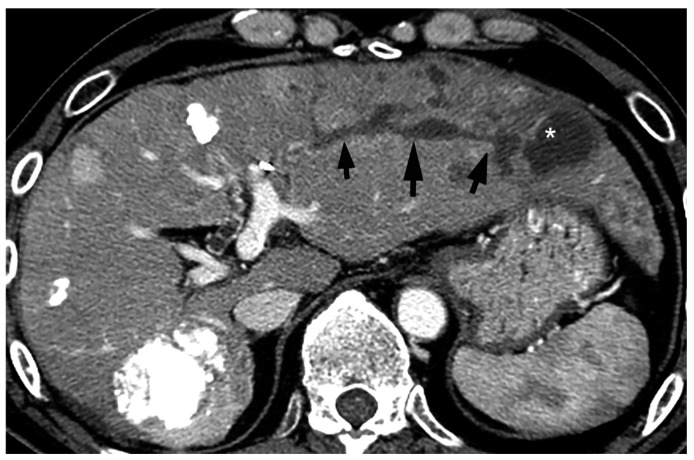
Portal phase image of contrast-enhanced CT of BDN and the biloma after transarterial therapy. A hypodense tubular structure (arrows) is connected to the large cystic lesion (*) in the lateral segment of the liver. The tubular structure is BDN of the anterolateral segment (S3) and the cystic lesion is biloma.
